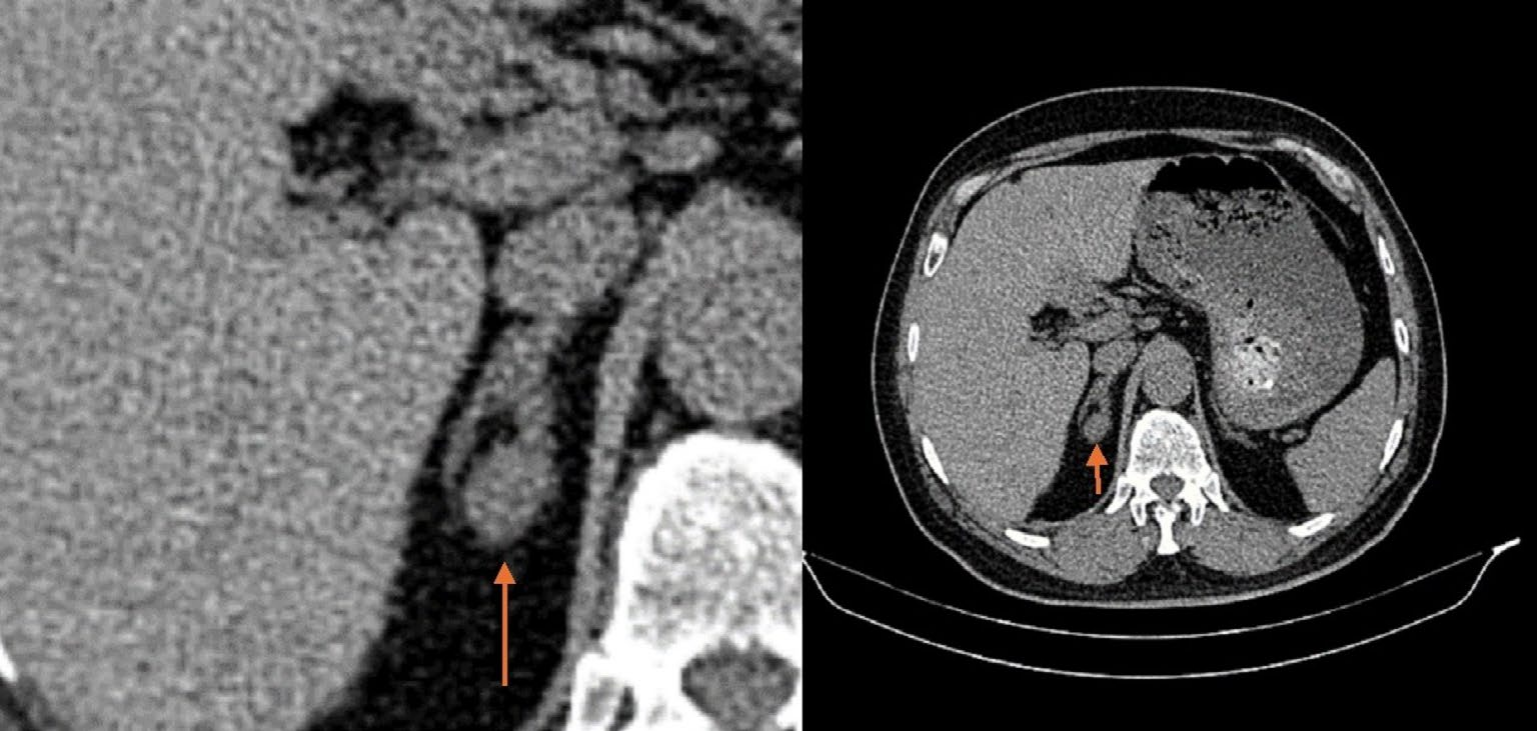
Represents the patient's abdominal CT scan showing a mass in the right adrenal gland (orange arrow).
